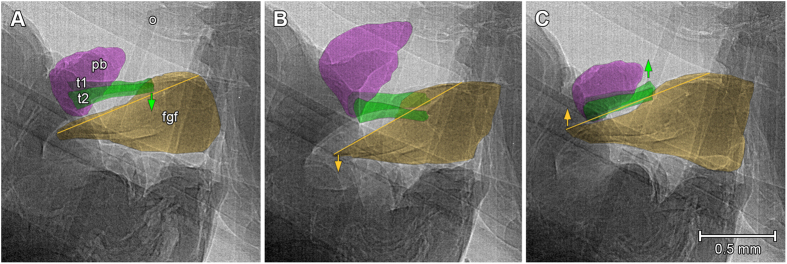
Rhythmic titillator movements of the male (left) open the female’s genital fold (fgf, on the right), revealed by X-ray imaging. The images were extracted from the video, which was recorded at 50 fps, 3.6x magnifications, and 3.06 μm pixel size. The external parts of the titillators are colored in green, the titillator basis is concealed within the male’s abdominal tissues. (A) The male’s titillators (t1 and t2, green) contact the female’s genital fold (fgf, yellow, the yellow line indicates the aperture of the fgf) and begin to push it down (green arrow). (B) The female’s genital fold opens wider (yellow arrow, the slope of the yellow line becomes steeper), while the titillators push it downwards. In the meantime, the male’s phallobasis (pb, purple) is touching the female’s ovipositor (o). (C) The titillators are retracted (green arrow) and the female’s genital fold is moving upwards in its initial position (yellow arrow, the slope of the yellow line levels out).
